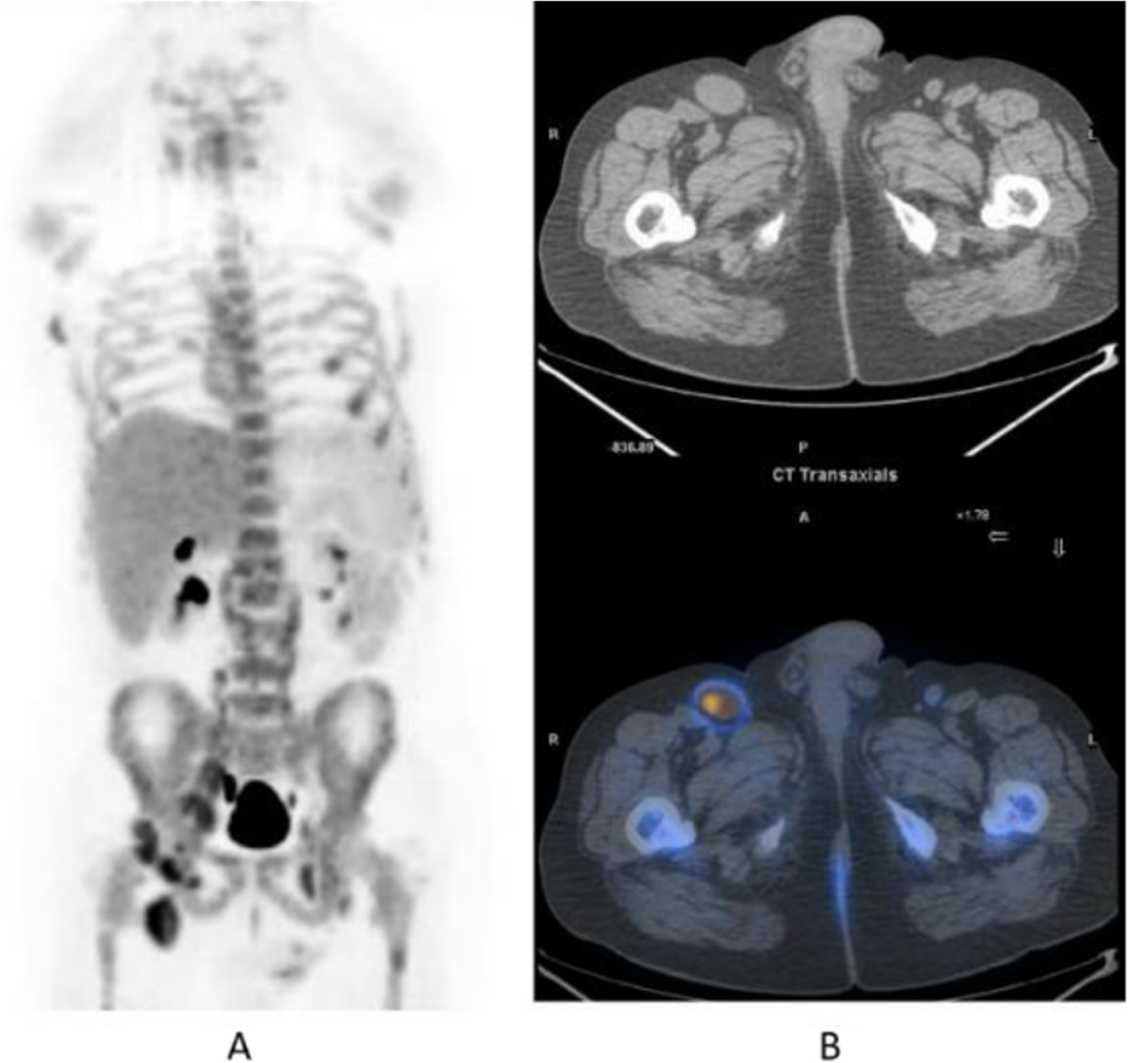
^18^F-FLT, non-Hodgkin lymphoma, staging. *Clinical history*: 52 y.o. patient with non-Hodgkin lymphoma at presentation. *PET/CT findings*: intense tracer uptake in enlarged inguinal and right iliac chain nodes. a MIP. bCT and fused images
